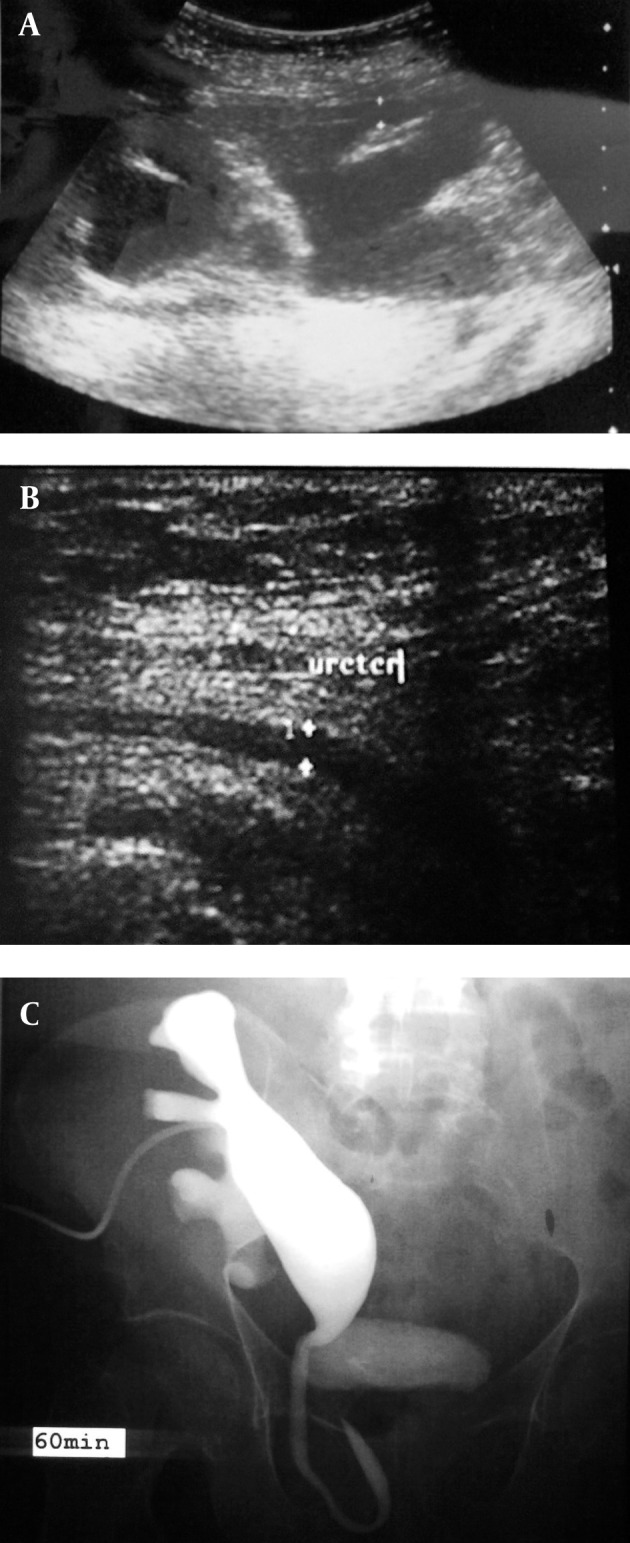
A 50-year-old man with allograft kidney transplant presenting with renal failure. A, Ultrasonography shows severe hydronephrosis of the transplant kidney. B, Dilated ureter in the inguinal canal is evident on ultrasonography. C, Nephrostogram shows the dilated pyelocaliceal system and a ureteral loop in the inguinal canal. The ureterovesical junction is not well visualized.
